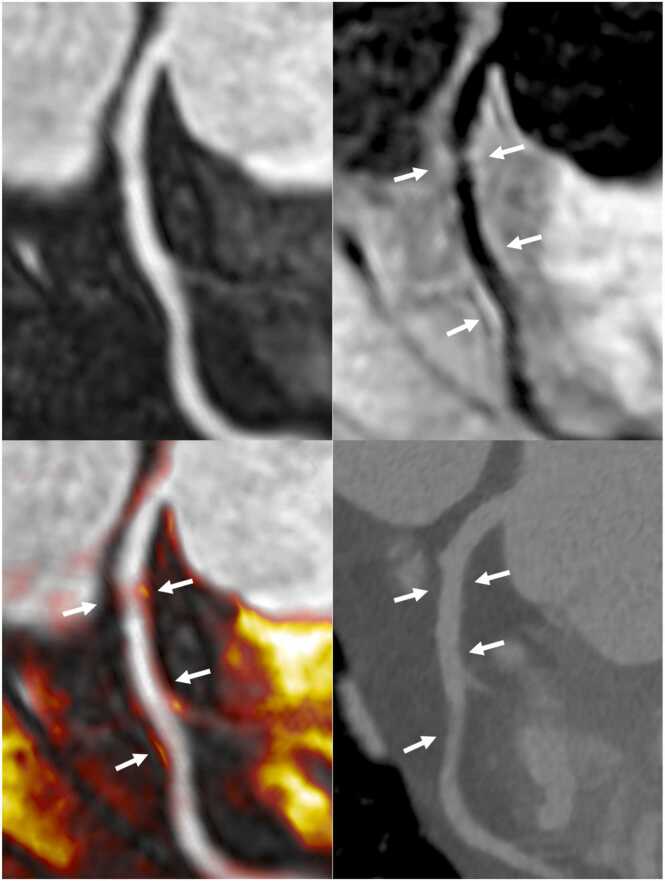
Multiplanar reformats of iT2prep-BOOST and CCTA imaging of a patient with CAD in the proximal and mid-LAD artery. Top left: BOOST bright-blood image demonstrating the coronary lumen; top right: BOOST black-blood image demonstrating the vessel wall; bottom left: fusion image of bright- and black-blood BOOST; bottom right: corresponding CCTA image. White arrows indicate the location of atherosclerotic plaques in the proximal and mid-LAD, which appear hyperintense on the black-blood image and correspond to non-calcified lesions seen on CCTA. *CCTA* coronary computed tomography angiography, *CAD* coronary artery disease, *LAD* left anterior descending, *BOOST* bright‐blood and black‐blOOd phase SensiTive
